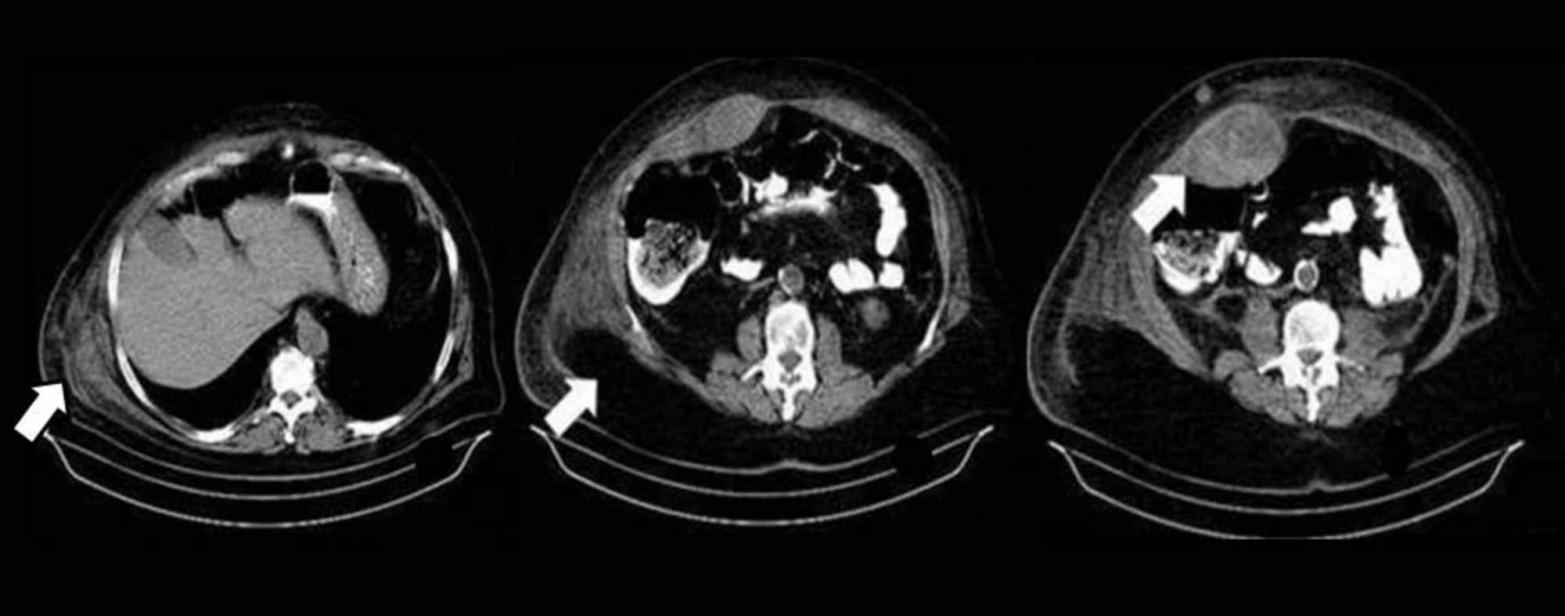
Computed tomography: giant bilobar RSH extended to internal and external oblique muscles, right transversus abdominis muscle, and subcutaneous fat tissue
